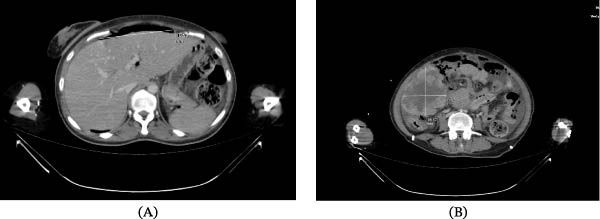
(A) Contrast‐enhanced axial abdominal computed tomography (CT) demonstrating free intraperitoneal air (arrow). (B) A large heterogeneous subhepatic mass with central necrosis measuring ~10 cm × 8 cm (arrows).
